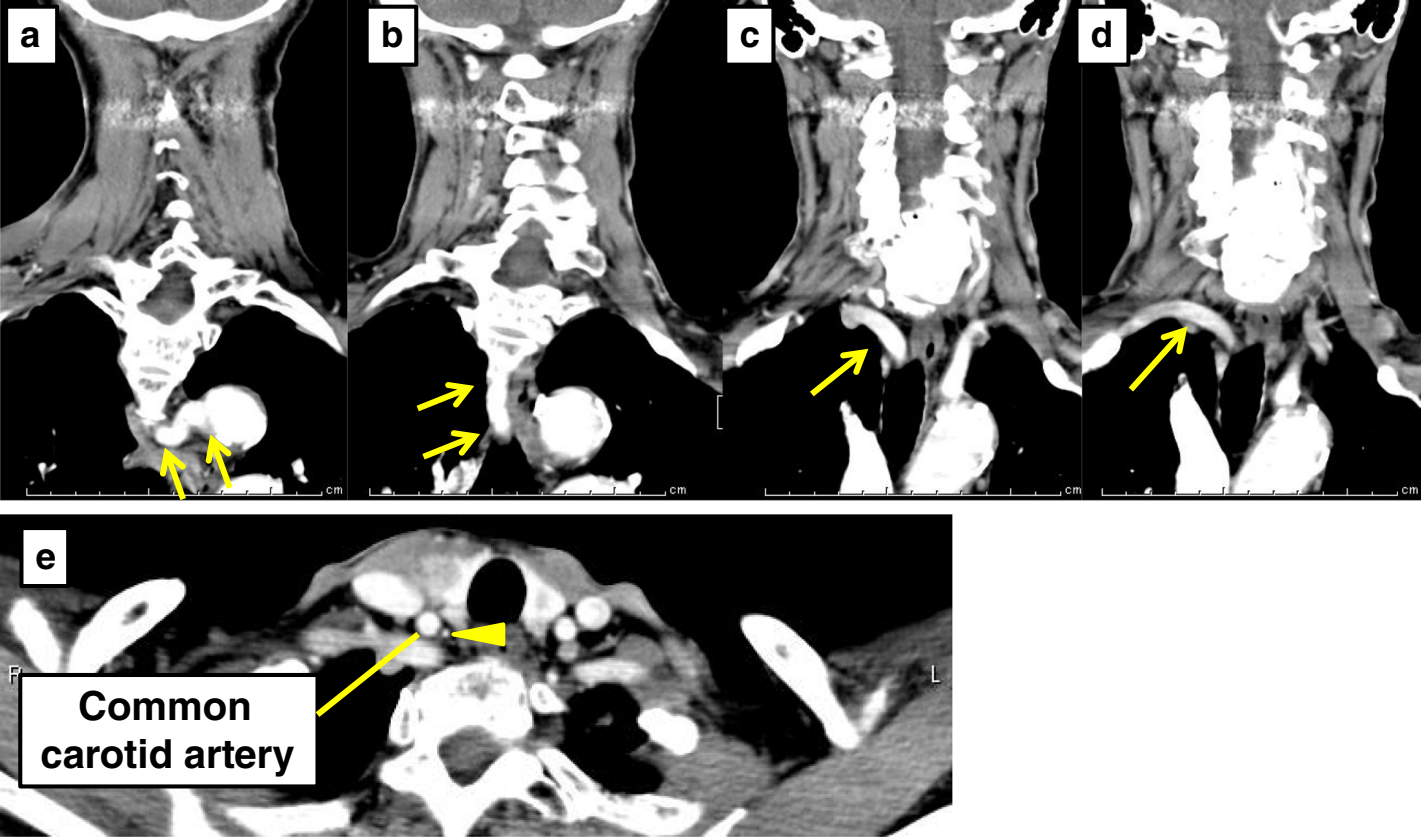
Preoperative enhanced CT scan of the neck and chest of case 3. a–d CT revealed an aberrant right subclavian artery (*yellow arrow*). e An artery ascending medial to the common carotid artery was thought to be a right vertebral artery (*yellow arrowhead*)
